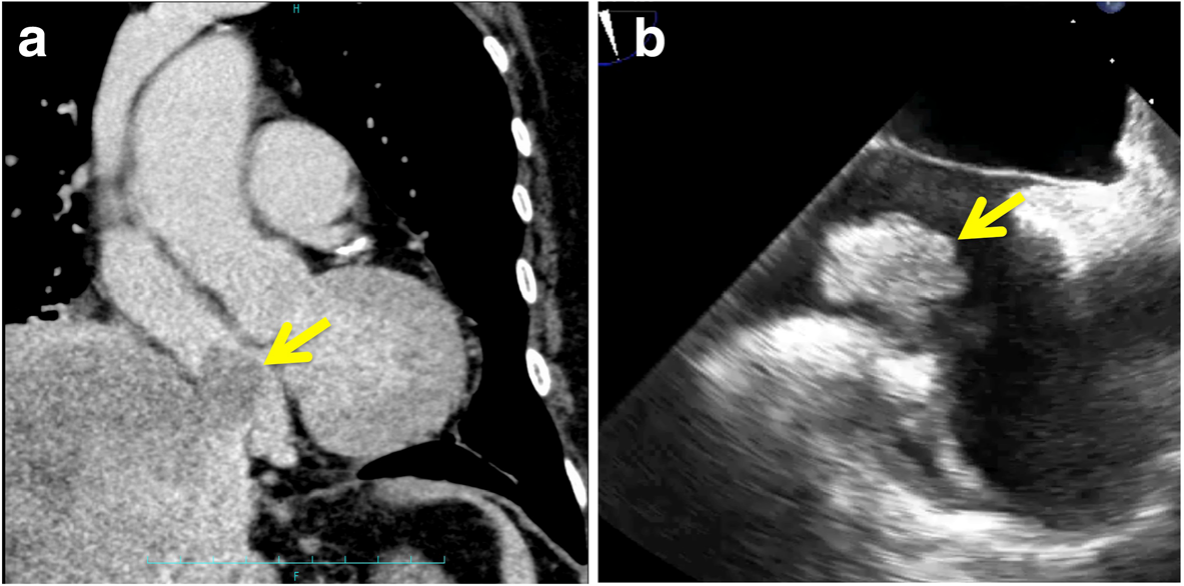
CT showed a tumor thrombus in the right atrium (a, arrow). The tumor thrombus moved with the heartbeat and reached the tricuspid valve on transesophageal ultrasound (arrow) (b)
